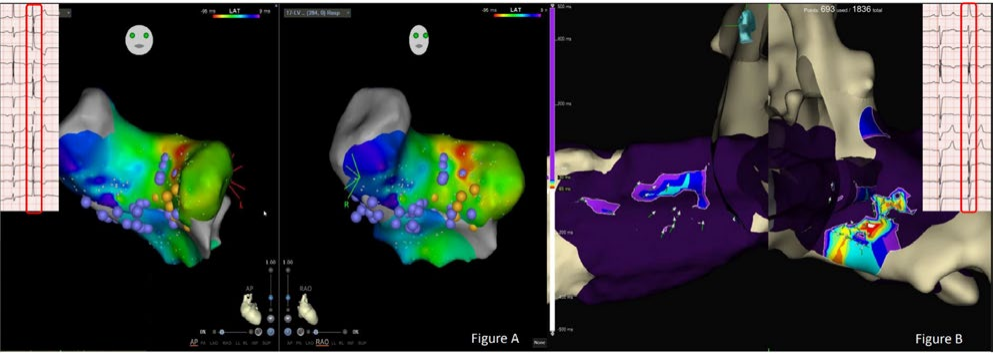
## INTRA‐PROCEDURAL ECHOCARDIOGRAPHY AND MANDATORY PHYSICIAN REFRESHER TRAINING REDUCES COMPLICATIONS FOR LEADLESS LEFT VENTRICULAR ENDOCARDIAL PACING
